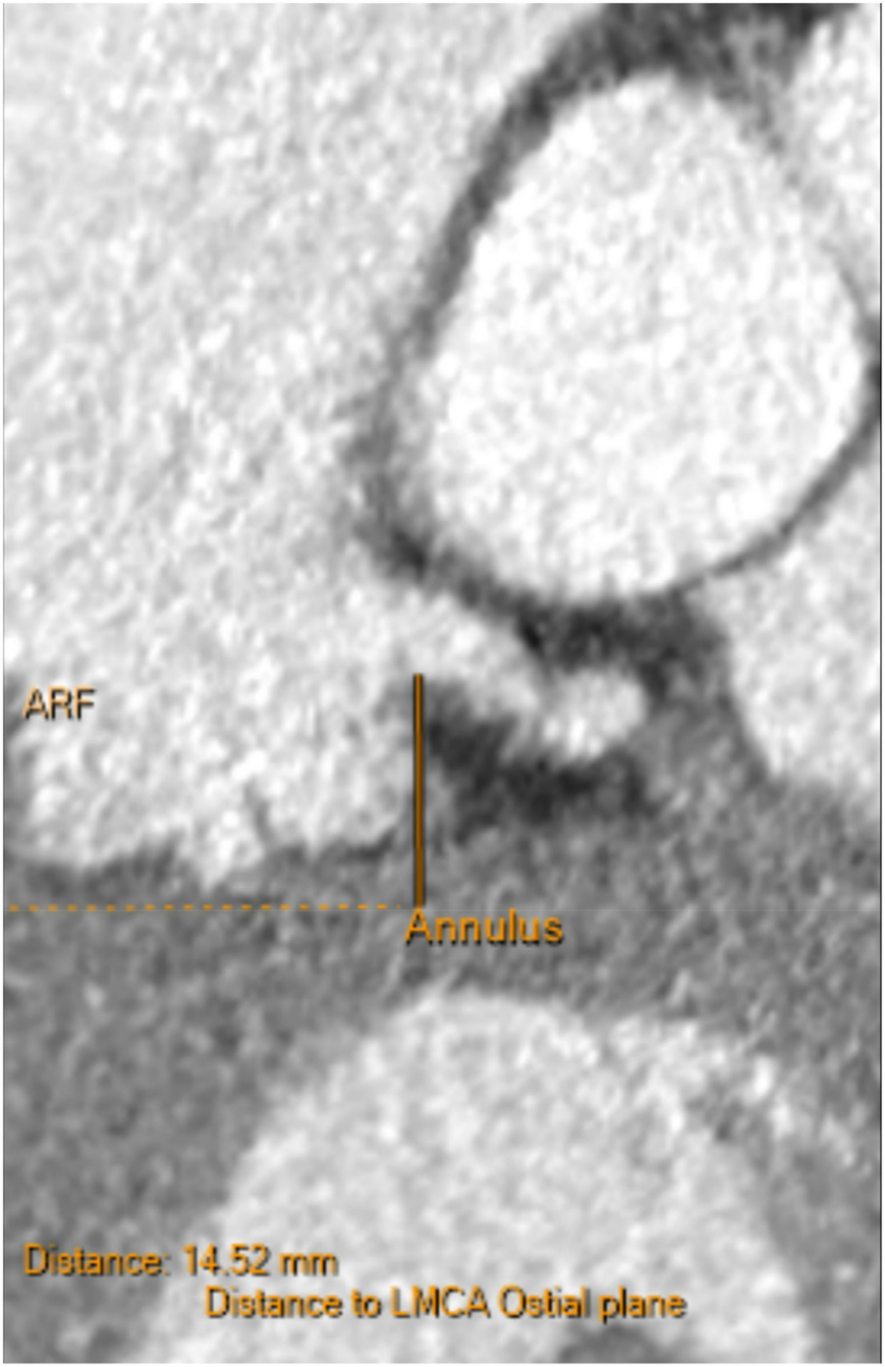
Pre-procedural CT demonstrating the left main coronary ostium 14.5 mm above the annular plane, confirming adequate clearance and favorable anatomy for TAVR. These measurements help exclude low coronary height as a mechanism for later ischemic events.
